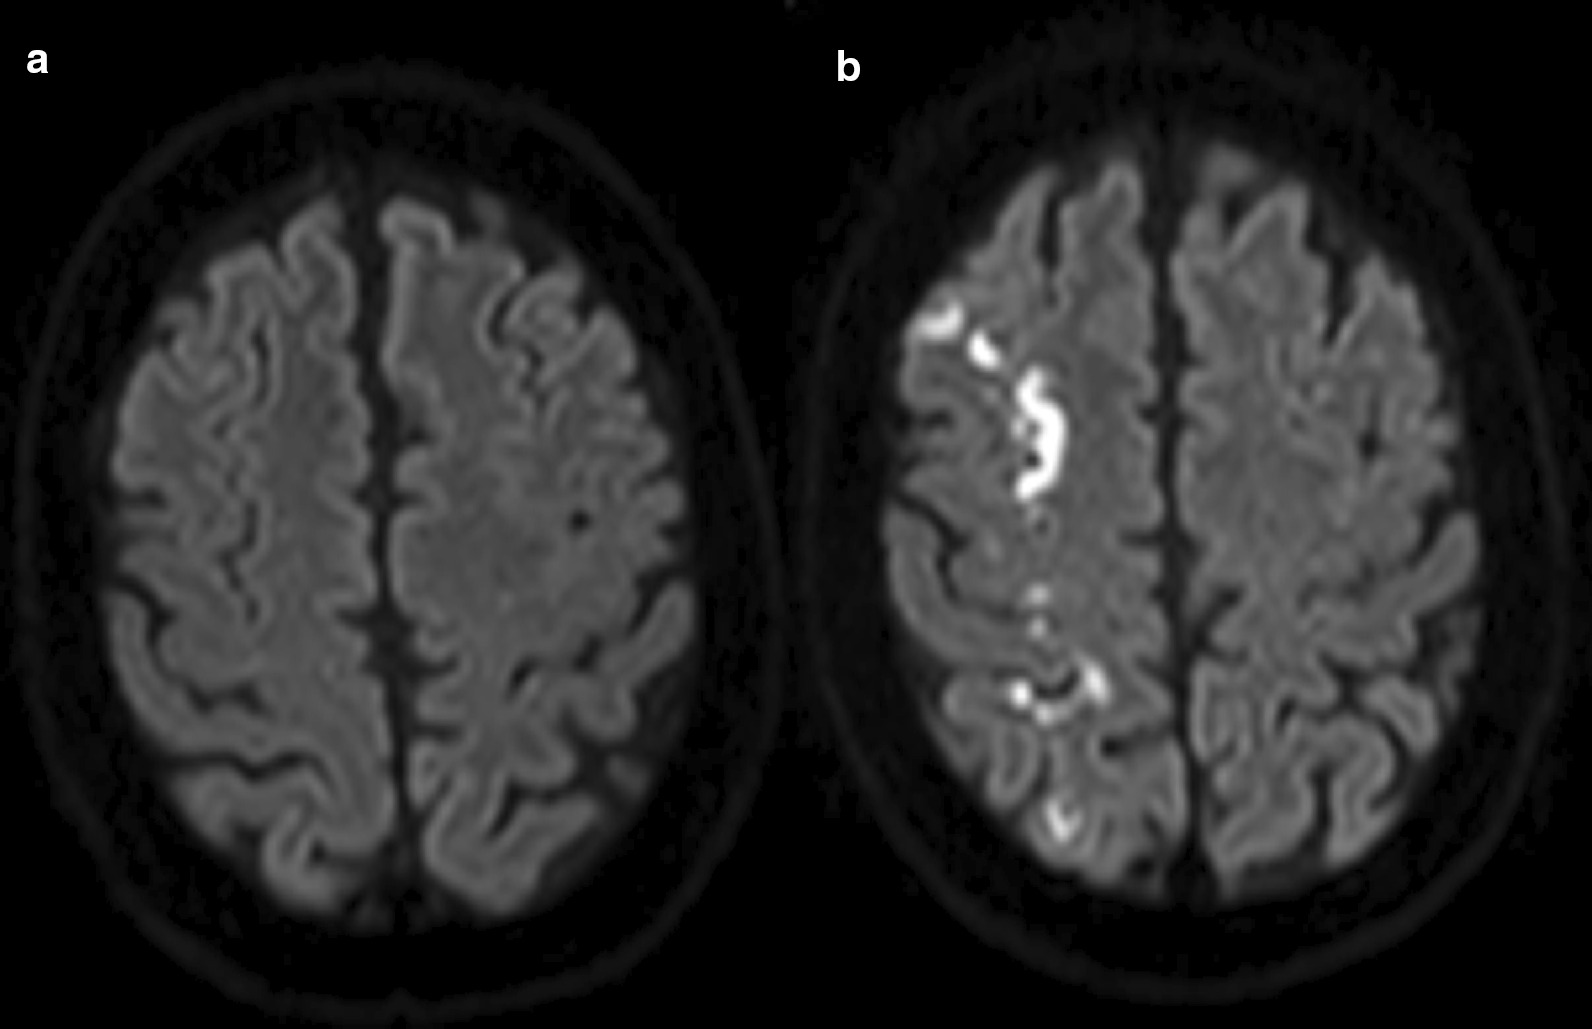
a Diffusion-weighted imaging (DWI) on day of admission. b DWI after neck massage and asystole. With permission of Prof. Ringelstein, Department of Radiology and Neuroradiology, Kliniken Maria Hilf
